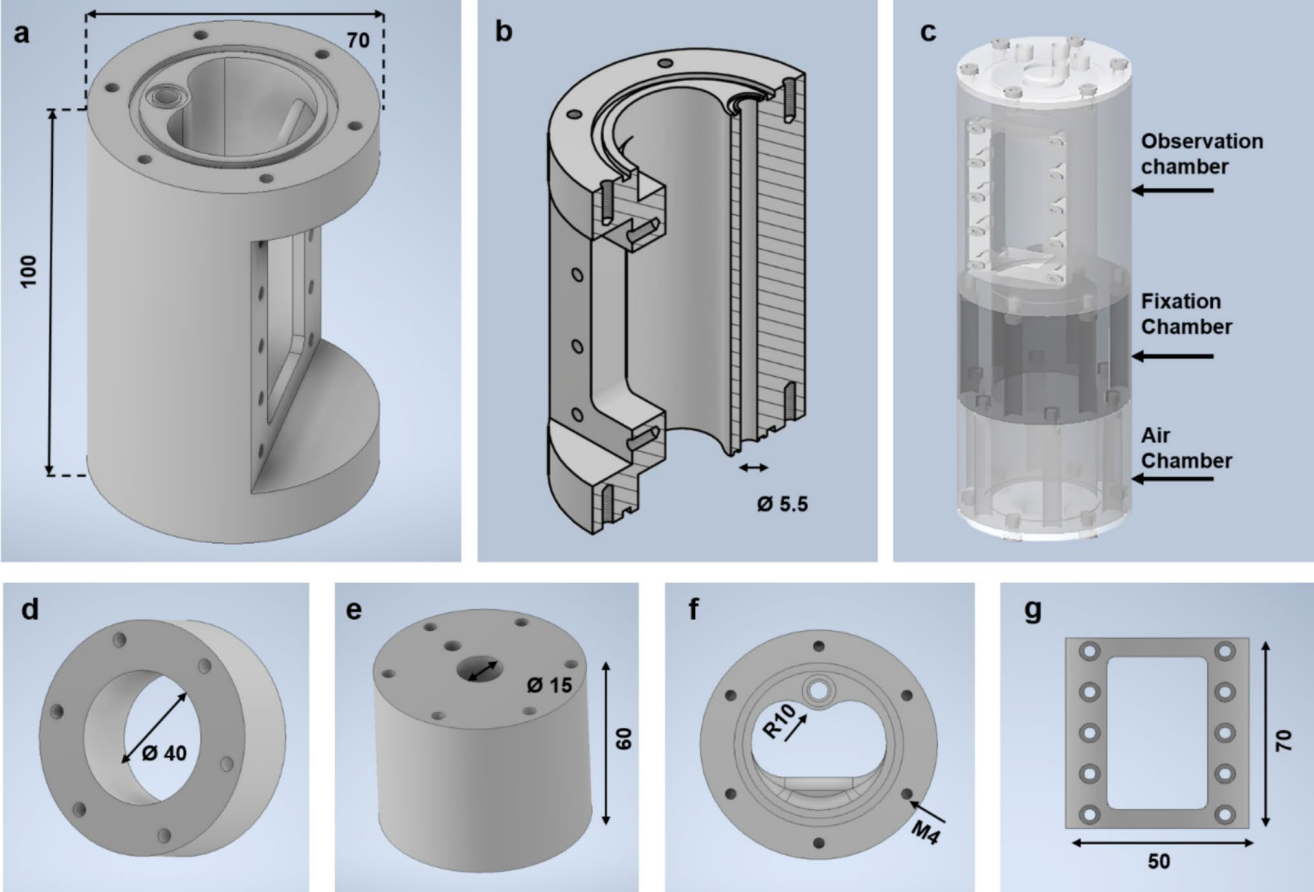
Computer-aided design of the bioreactor chambers. a The observation chamber with an integrated backflow. b Side view of the observation chamber showing the vertical cross-section. c Assembly of all chambers of the bioreactor. d Side view of air chamber. e Side view of fixation chamber. f Top view of the observation chamber. g The ultrasound window cap. All the dimensions are in mm
